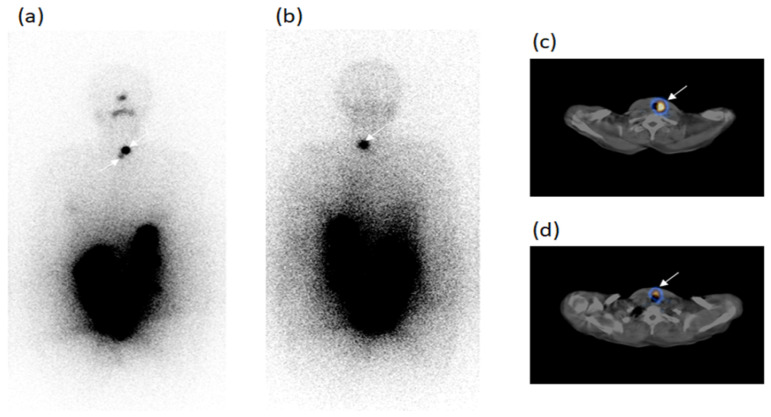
A 60-y-old female patient (case n. 13, Table 4) submitted to total thyroidectomy for multifocal-bilateral papillary carcinoma, tumor diameter 15 mm, at the first radioiodine ablation.^131^I-WBS after therapeutic radioiodine dose, in both anterior (a) and posterior (b) views evidenced two radioiodine-avid foci of different activity in the median region of the neck classified as tissue residues. Fused SPECT/CT images in transaxial slides (c,d) also identified the two foci evidenced by WBS confirming residual origin. Both WBS and SPECT/CT excluded the presence of foci doubtful of malignant lesions. Thyroglobulin levels in hypothyroidism condition: 4.2 ng/mL. AbTg: absent.
